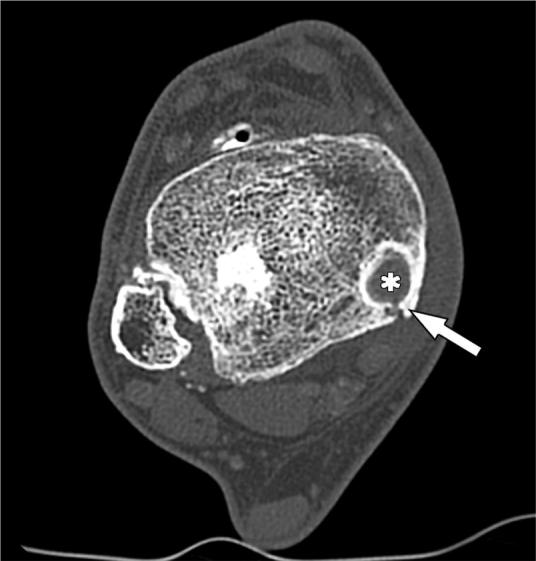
Ax CT scan, showing the tibialis posterior tendon (*) in a medial retromalleolar bone tunnel and the healed posterior malleolus fracture (red line).
